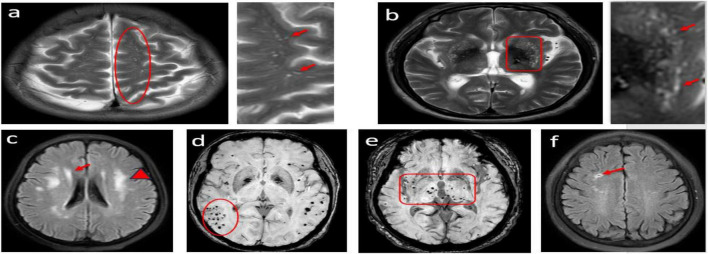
Cases of MRI findings for lesions related to small vessel disease; (a) Axial T2-weighted MRI showing punctuate and linear hyperintensities characteristic of enlarged perivascular spaces (EPVS) in the centrum semiovale. (b) punctuate hyperintensities characteristic of EPVS in the basal ganglia. (c) Deep white matter hyperintensity (WMH) graded as 3 (red arrowheads) in a patient who also has periventricular WMH (graded as 2, red arrows). (d,e) different topographic distributions cerebral microbleeds (lobar/deep). (f) lacunes of presumed vascular origin.
